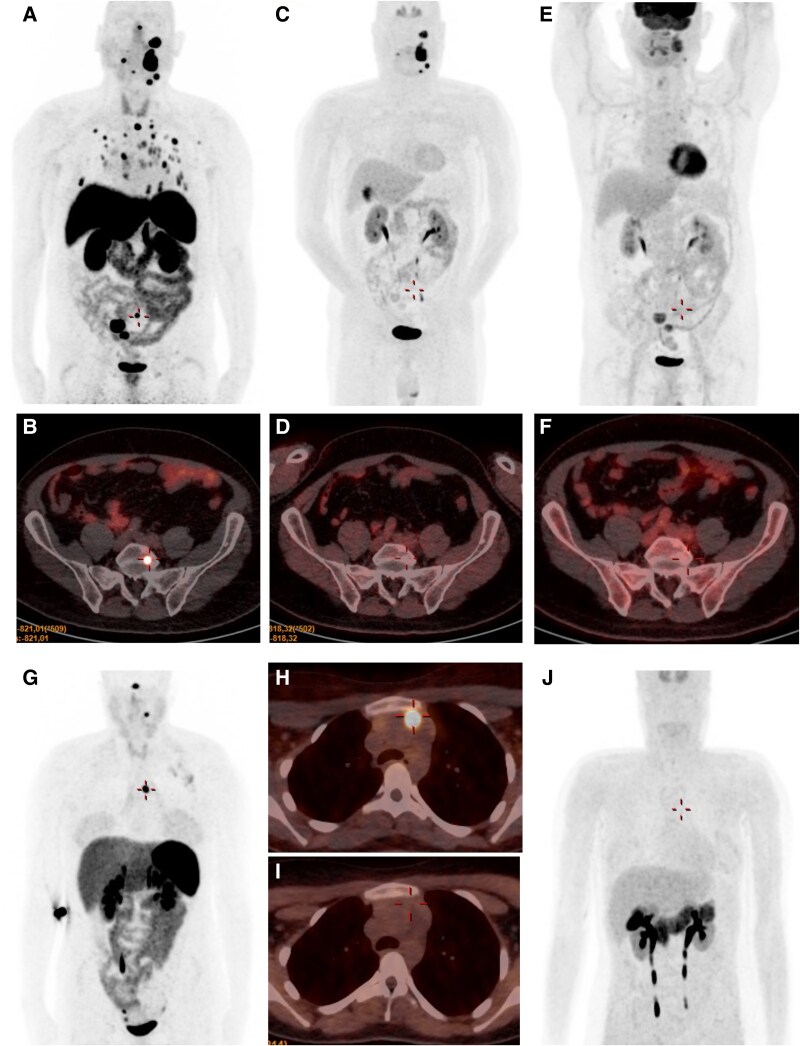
Comparative functional images of patients with extracervical and malignant paragangliomas associated to the *SDHAF2* gene. A 54-year-old man with multifocal head and neck paragangliomas along with bone and lung metastases according to [68Ga]Ga-DOTA-TOC PET/CT (A, B). [18F]DOPA PET/CT (C, D) and ^18^F-FDG PET/CT (E, F) failed to show focal uptake of the metastatic lesions. A 14-year-old woman with intense focal [68Ga]Ga-DOTA-TOC uptake at the head and neck (G) and mediastinal level (G, H) and negative [18F]DOPA PET/CT (I, J).
